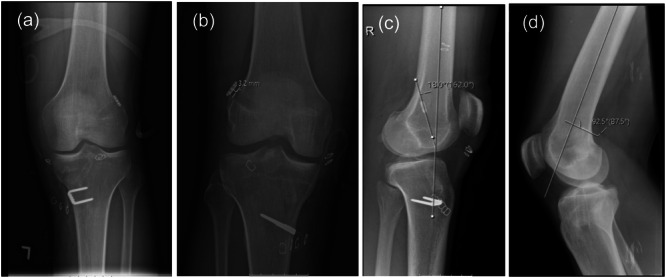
(a–d) Radiographic measurements. (a) An antero‐posterior (AP) X‐ray of unmeasurable distance to the lateral femoral condyle (LFC) using a fixed‐cortical suspension device, calculated as 0 mm. (b) An AP x‐ray demonstrating 3.2 mm button‐LFC distance using fixed‐cortical suspension device. (c) Lateral X‐ray showing the angle between the button orientation and the femoral anatomical line, calculated as 18.0°. (d) Lateral X‐ray demonstrating the button location in the sagittal plane of the femur, which is divided into three equal sections (anterior, middle and posterior). The button is located in the posterior third in this patient.
